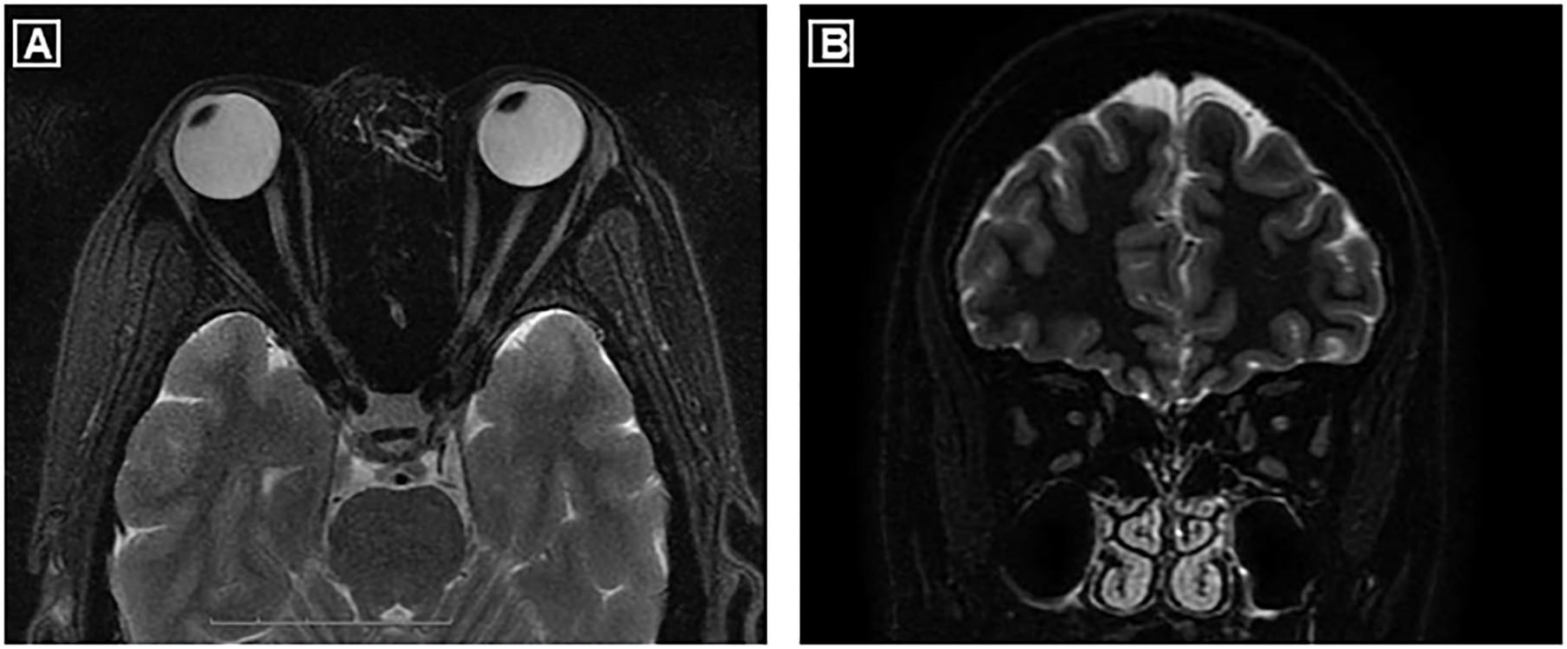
Axial (A) and coronal (B) MRI scans of optic nerves showed bilateral atrophy.
